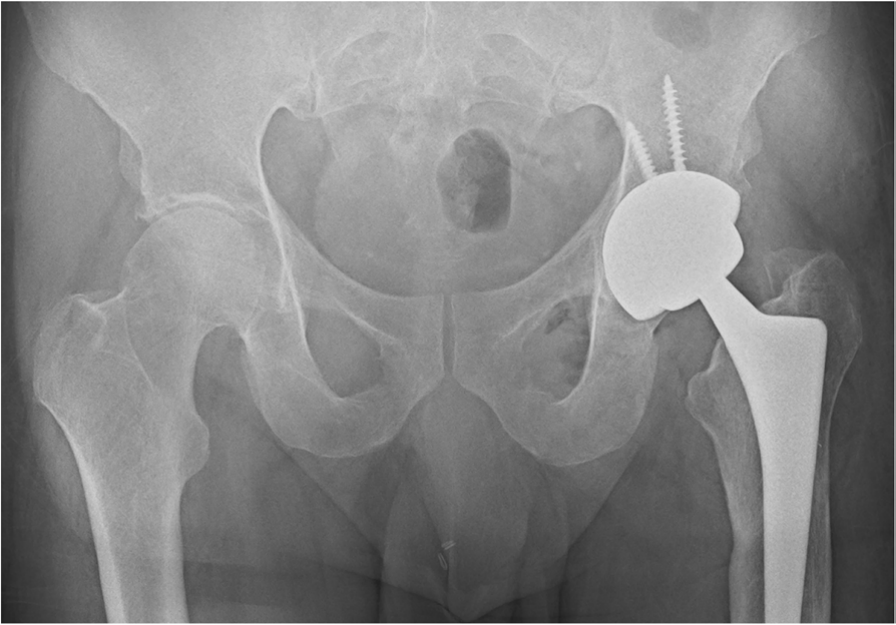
Post-operative pelvis radiograph showing the revised acetabular component 2 mm larger than the explanted Maxera cup. Modular cup with ceramic acetabular bearing (40 mm) and supplemental fixation with 2 screws
